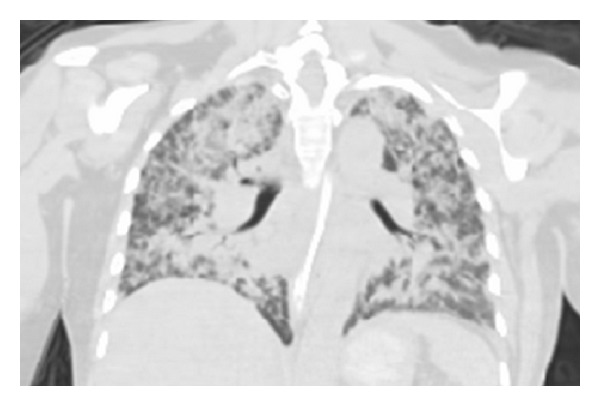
CT thorax demonstrating diffuse airspace opacities involving bilateral lung fields.
